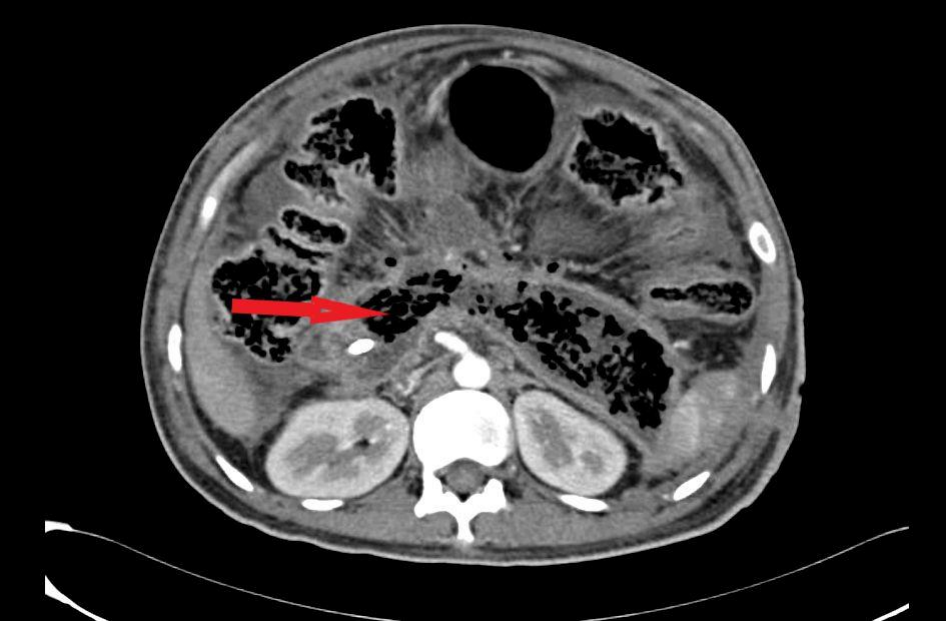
Contrast-enhanced computed tomography (CECT) image showing pancreatic necrosis. The red arrow shows less enhancement suggestive of necrosis.
